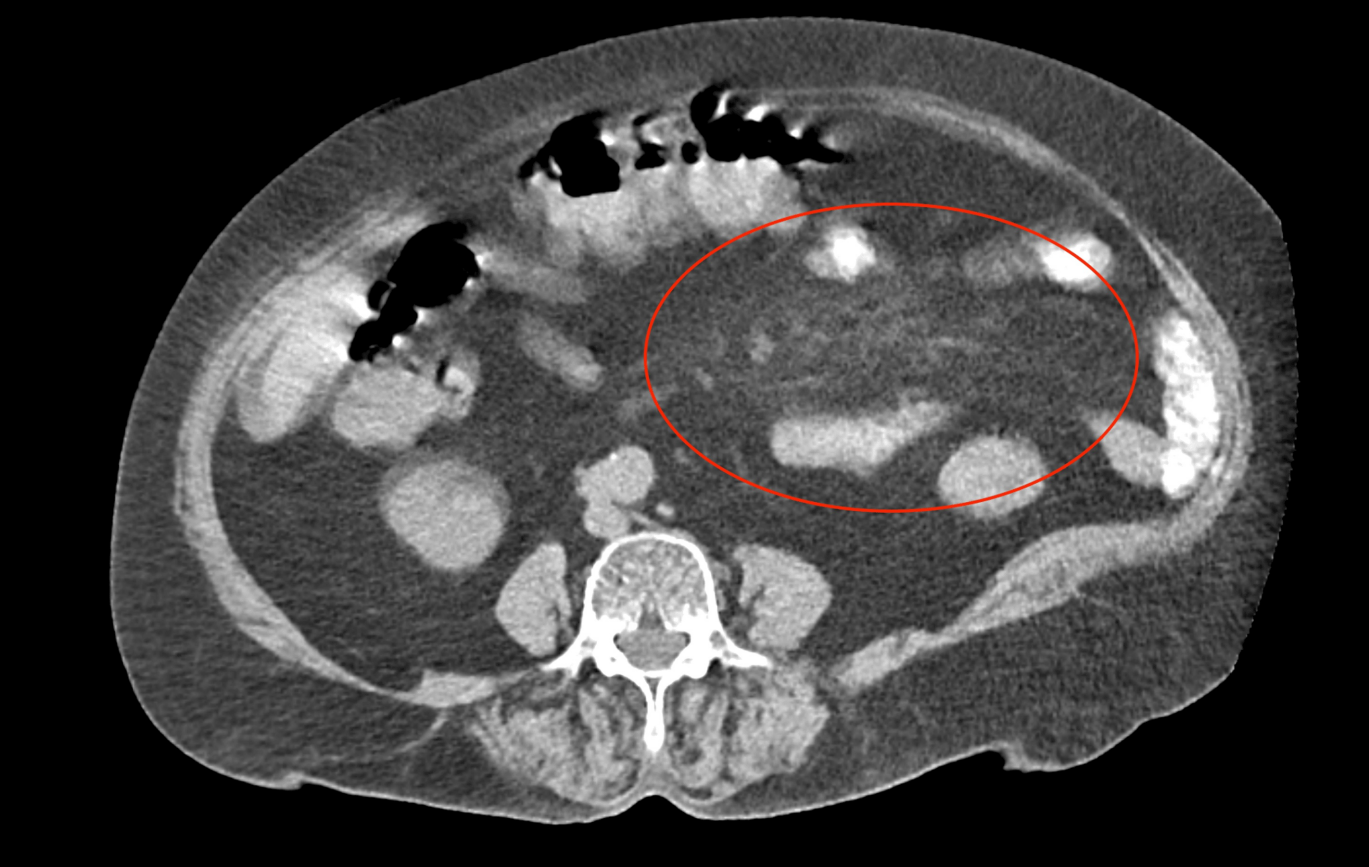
CT of the abdomen demonstrating the “misty mesentery” appearance Transverse view showing increased attenuation of mesenteric fat with ill-defined borders.
